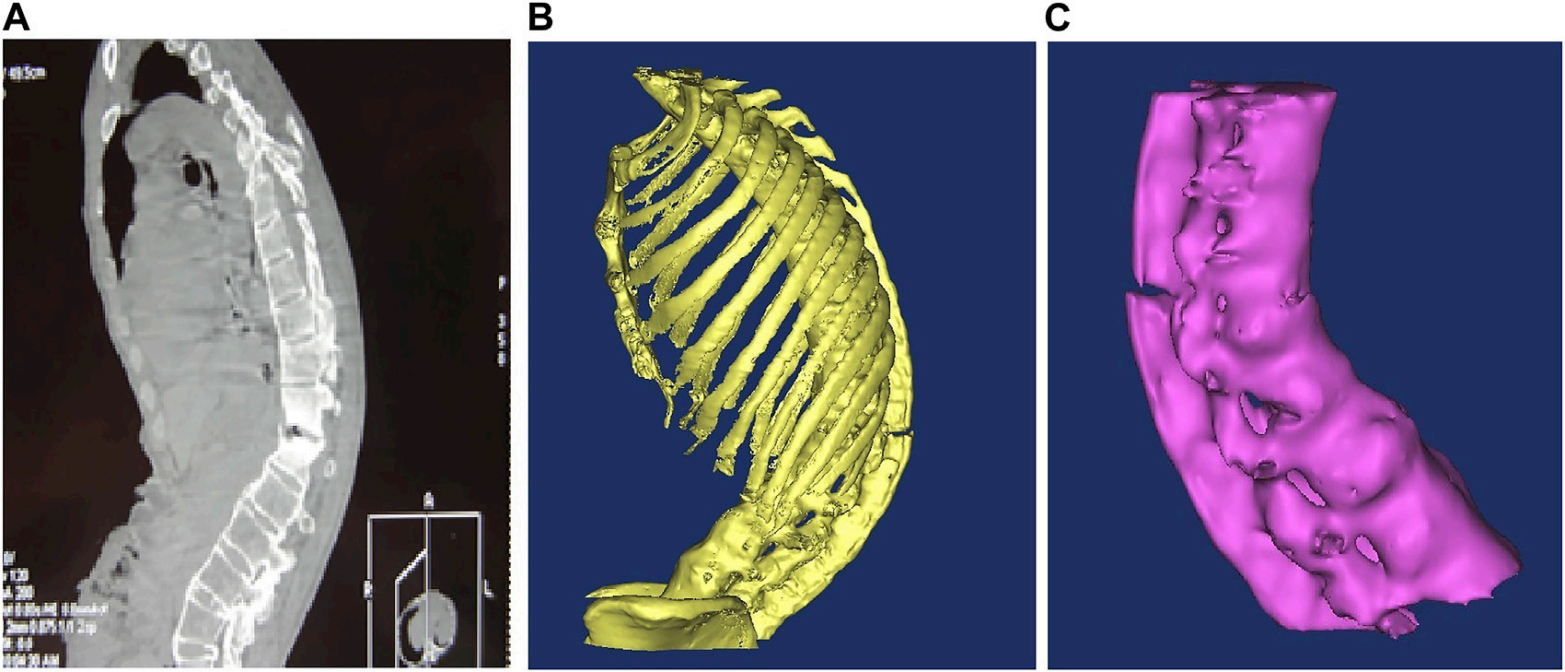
(A) Ankylosing spondylitis CT scan lateral radiographs; (B) 3D model after noise removal and boundary smoothing; (C) bone independent boundary modification was removed and T10-L4 segments were retained.
